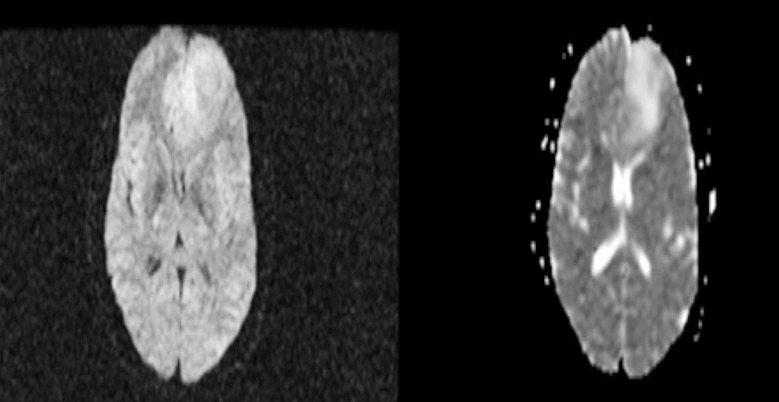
DWI and ADC of Diffuse Astrocytoma. The tumor in left frontal lobe show no diffusion restriction.
